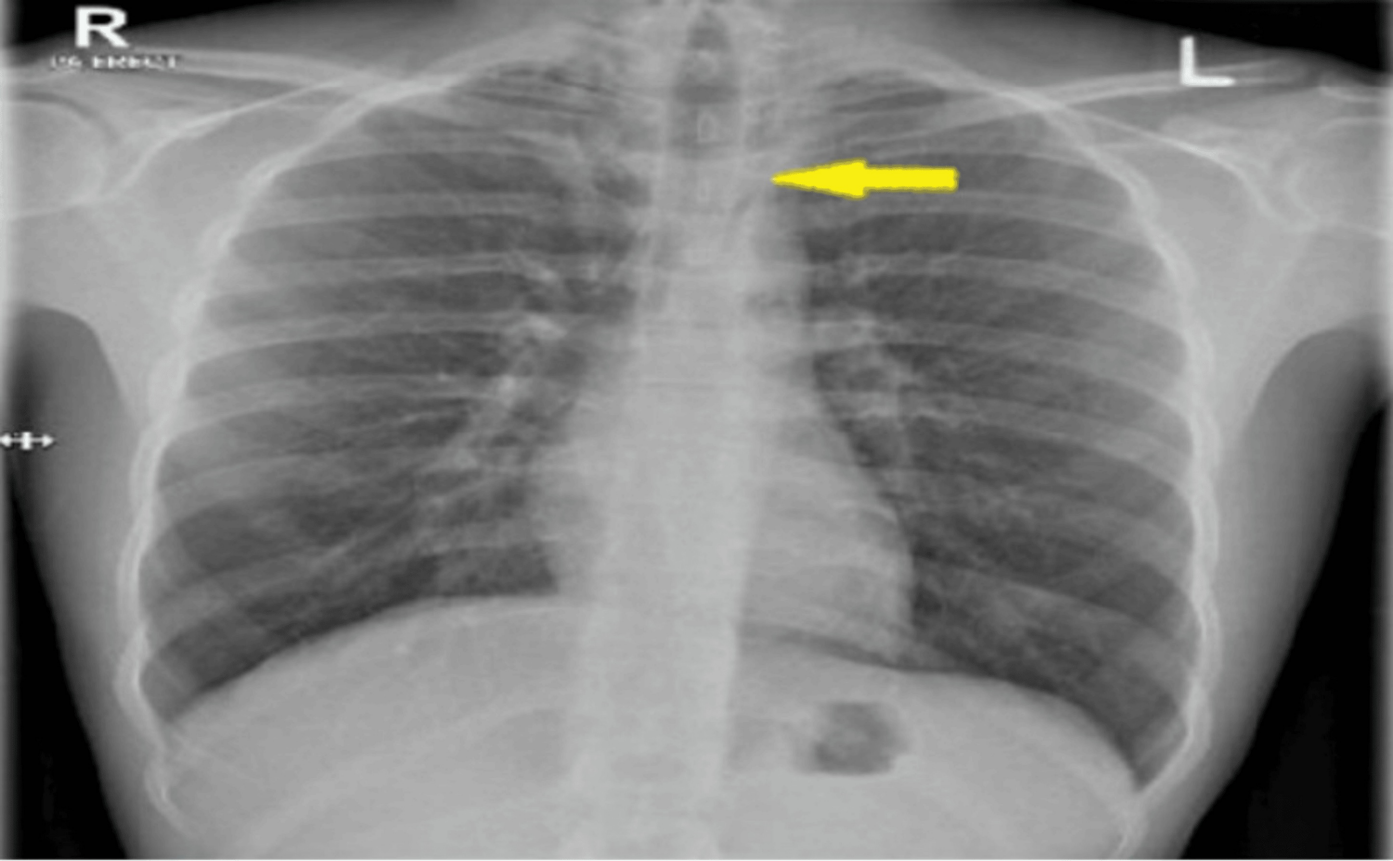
Supine chest X-ray showed air located at the mediastinum (pneumomediastinum) highlighted by the yellow arrow.
